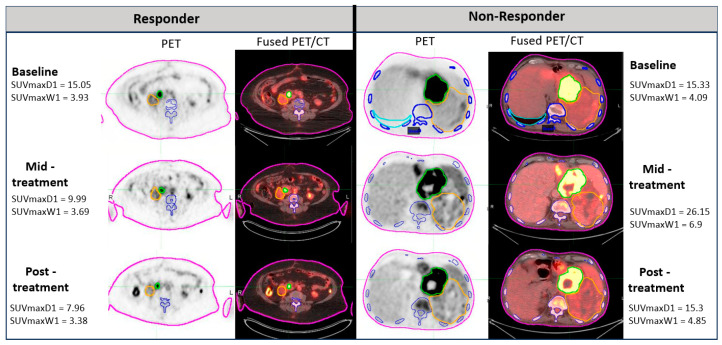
An example of images representing responder and non-responder patients with liposarcoma. Longitudinal axial PET and fused PET/CT images demonstrating the presence of abdominal DDLPS (“D” with green delineation) and WDLPS (“W” with orange delineation) tumors over the course of treatment. The yellow (orange) contours were manually drawn since the threshold segmentation would not pick up the WDLPS due to small differences between the tumor and the background. The DDLPS tumor demonstrated a significant decrease in ^18^F-FDG radiotracer activity (>50% of SUVmax decrease from baseline), as well as in volume in the responder. On the contrary, the DDLPS tumor ^18^F-FDG radiotracer activity increased in the non-responder. In both cases, there was no significant difference in ^18^F-FDG uptake in WDLPS tumors as a response to treatment. Delineation color legend: pink = body contour; blue = bone; sky blue = lung contour.
